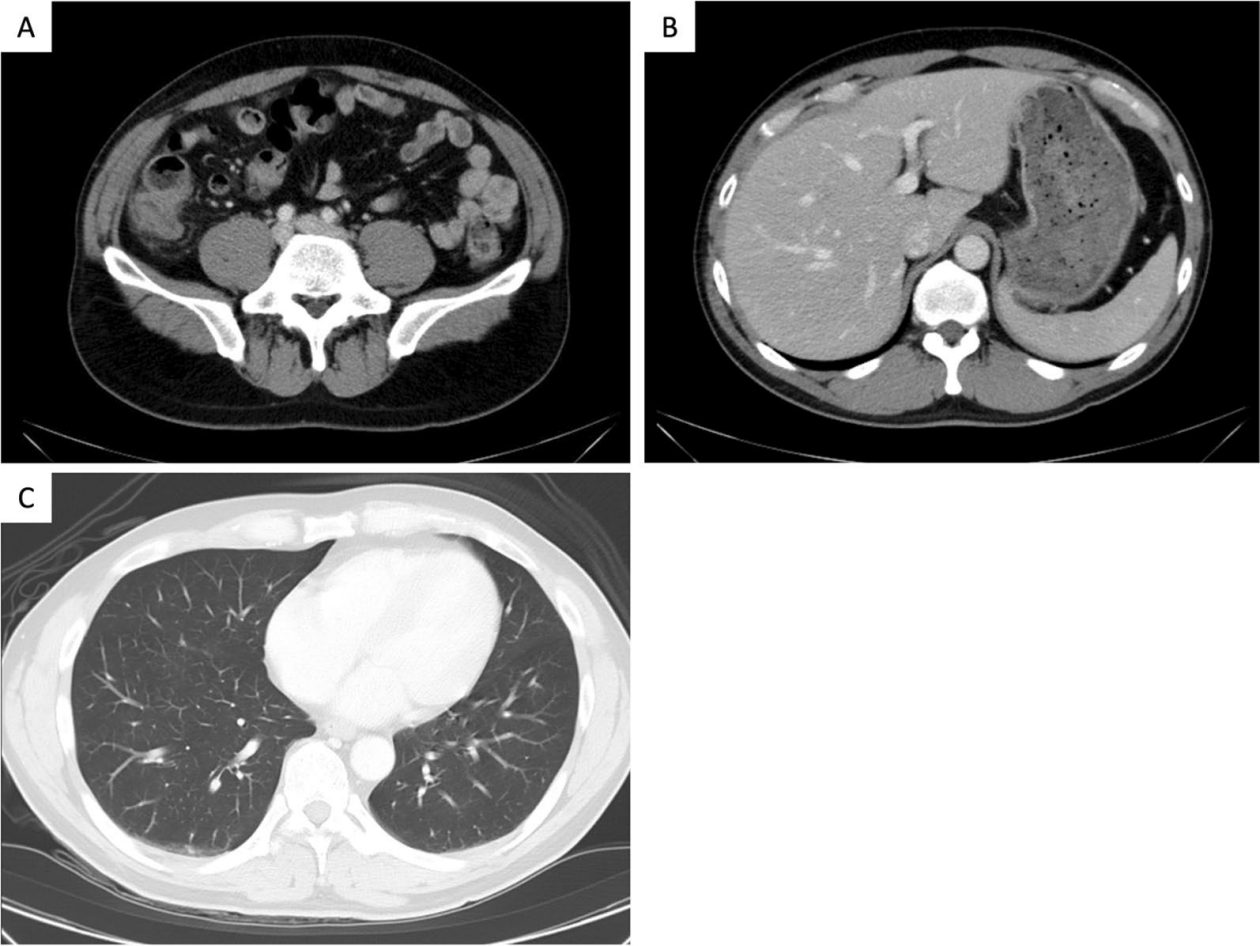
Preoperative CT showed no mass or enlarged lymph nodes. a There is not mass in the ileocecal area. b There is not hepatic mass. c There is not lung mass.
